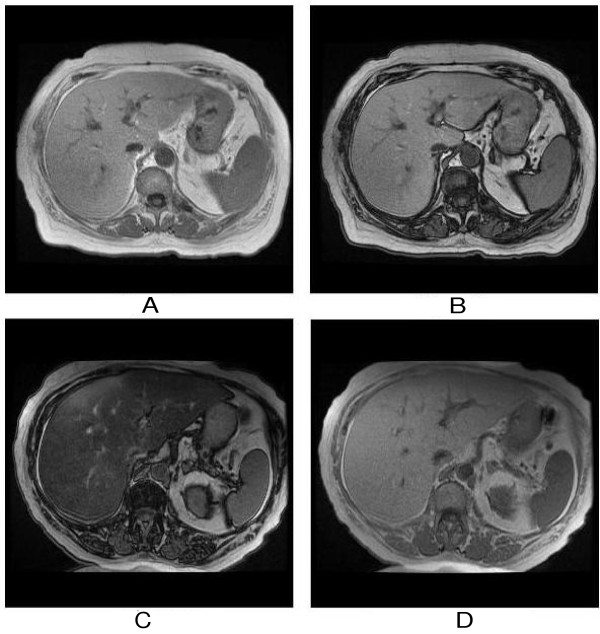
Capecitabine-induced hepatic steatosis in a 74-year-old woman. Dual-echo, chemical shift gradient-echo T1-weighted magnetic resonance images show no evidence of hepatic steatosis which is demonstrated by the signal intensity of the liver on the in-phase (TR/TE, 150/4.5) image (A) similar to that of the out-of-phase (150/2.3) image (B). Three-month follow-up magnetic resonance images after treatment with capecitabine clearly show newly developed severe hepatic steatosis which is seen as a drop of the signal intensity of the liver on the out-of-phase (150/2.3) image (C) compared with the in-phase (150/4.5) image (D).
